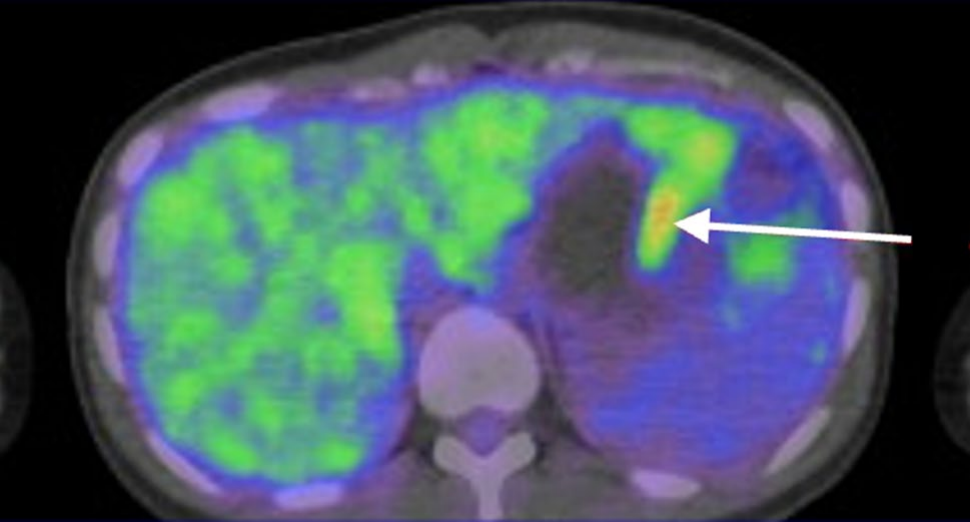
Positron emission tomography–computed tomography demonstrated 18F-fluorodeoxyglucose uptake only in the solid component (white arrow) of the pancreatic tail cyst, and maximum standard uptake values (SUVmax) was 2.94
